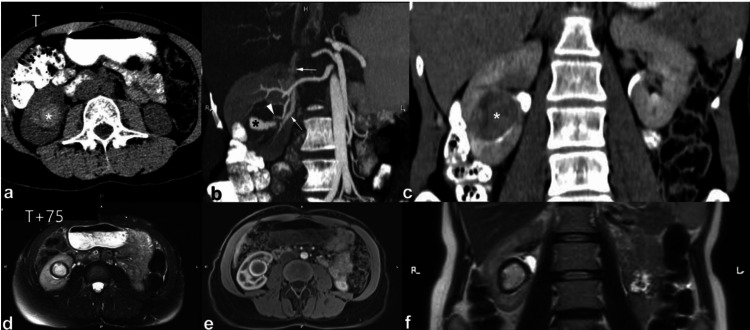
A comprehensive imaging assessment was conducted using multiple modalities, including non-enhanced CT (NECT) (a), contrast-enhanced CT (CECT) in the arterial phase with reconstruction in an oblique plane (b), CECT in the urographic phase with reconstruction in the frontal plane (c), MRI axial T2 half-Fourier acquisition single-shot turbo spin echo imaging fat suppression (HASTE FS) (d), MRI axial Volumetric interpolated breath-hold examination (VIBE) post-gadolinium in the arterial phase (e), and MRI coronal T2 half-Fourier acquisition single-shot turbo spin echo imaging (HASTE) (f). In the case of a 35-year-old woman who recently gave birth, abnormalities in renal arterio-venous communication with associated pseudoaneurysms were identified, denoted by black asterisks. Specifically, the feeding artery (arrowhead) and venous drainage (arrows) exhibited notable anomalies, indicative of the presence of pseudoaneurysms. Additionally, a lesion resembling a hematoma in the kidney region was observed, characterized by the presence of spontaneous hyperdensities compatible with acute hemorrhage. Following a 75-day interval, subsequent MRI imaging revealed a significant resolution of the malformation, indicating spontaneous regression. Moreover, the hematoma appeared to have diminished in size. This figure is a republished image, permission was obtained from the original publisher, https://epos.myesr.org/poster/esr/ecr2018/C-2802
